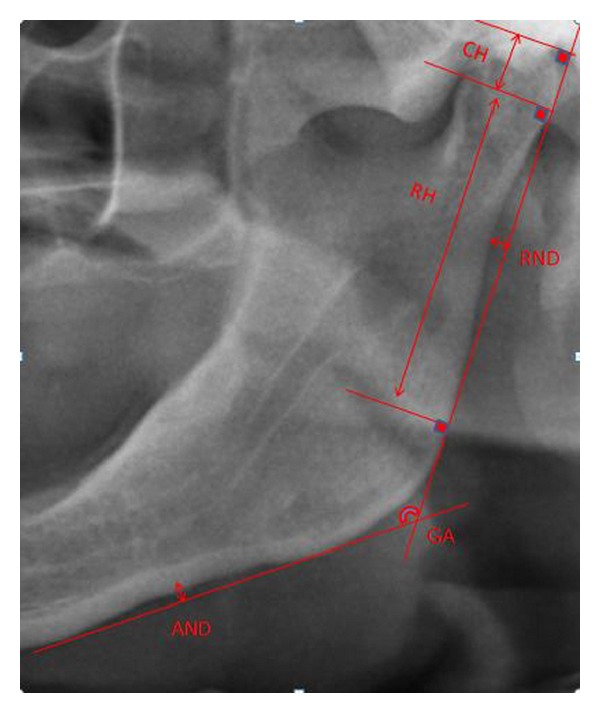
Measuring method used for the gonial angle, ramus height, condylar height, antegonial notch depth, and ramal notch depth on panoramic radiograph.
